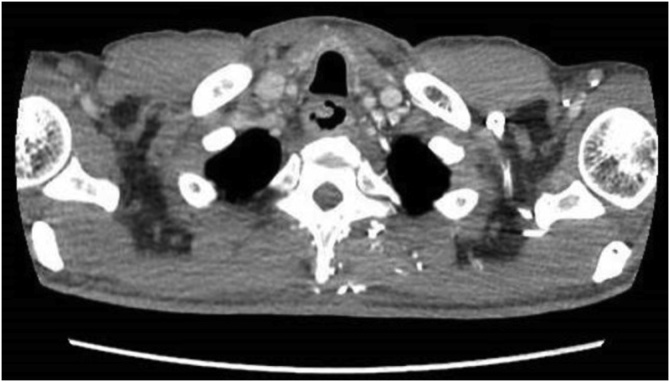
Axial view of CT showing the polyp stalk arising from the right of the cervical oesophagus.
